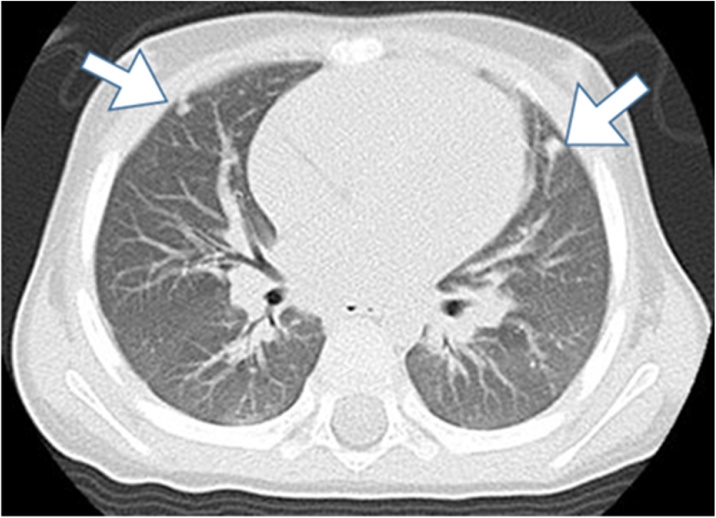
Chest computed tomography showing parenchymal nodules suspicious for metastases in both lungs.
